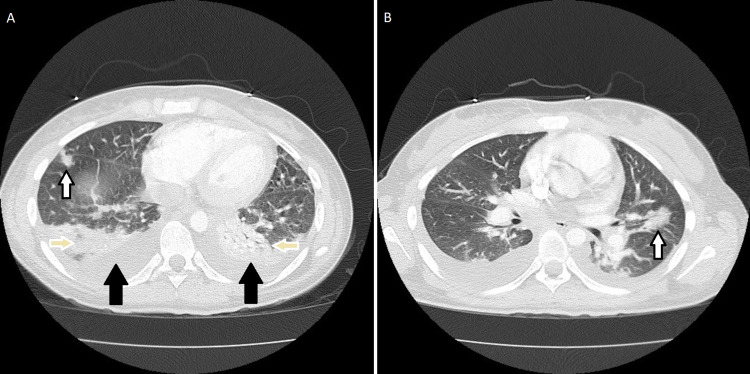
Chest CT with contrast demonstrating pleural effusion (black arrows), consolidation (yellow arrows) and nodular opacities (white arrows).
